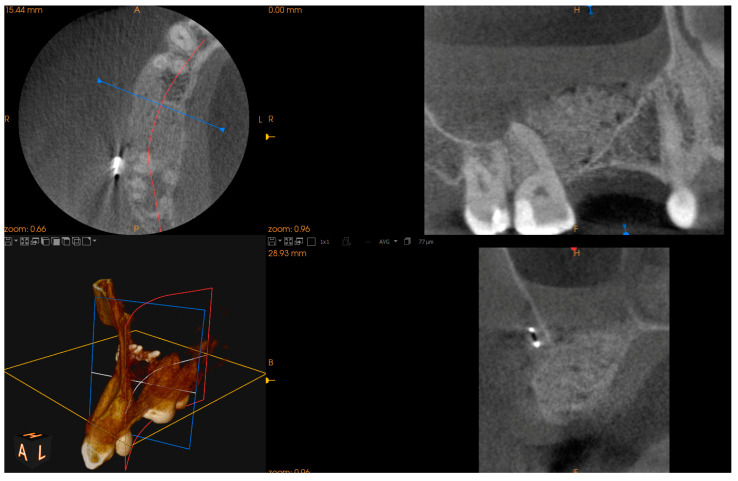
Screenshot from post-op CBCT scans with visible xenograft and a flat level of liquid in the maxillary sinus lumen due to present perforation.
